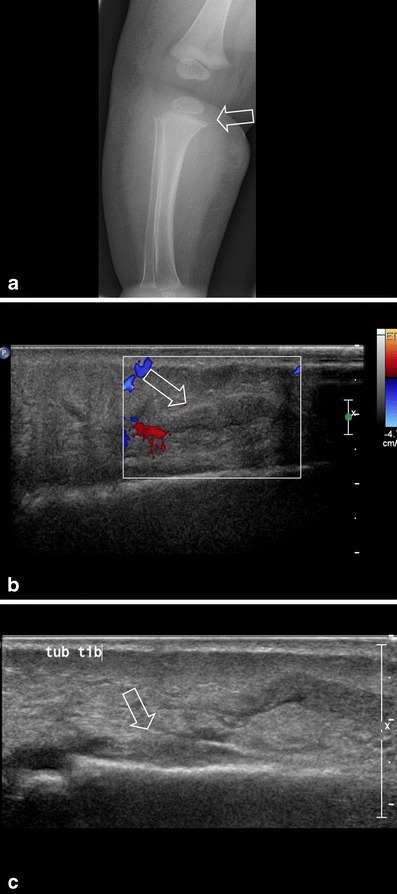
a A 3-month-old girl with tenderness of the right leg and fever. Imaging shows soft tissue swelling around the tibia. The periostium of the proximal metaphysis is slightly irregular (*arrow*). b Ultrasound with Doppler shows infiltration of the subcutis, hyperaemia and a long subcutaneous fluid collection (*arrow*). c Subperiostal fluid collection/abscess (*arrow* shows uplifted periostium). Surgically the abscess was drained and the patient received antibiotics
